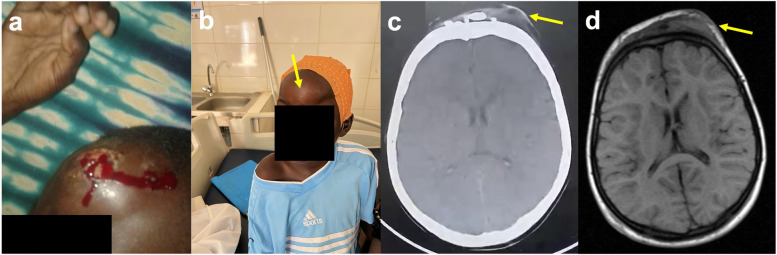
Preoperative findings. a Traumatic forehead injury sustained during drop attacks. b Forehead chronic traumatic scalp lesions resulted from repeated head impacts during drop attacks (yellow arrow). c CT demonstrated prominent forehead callosities (yellow arrow) without underlying structural lesion. d MRI showed forehead lesions (yellow arrow) but otherwise confirming the absence of focal pathology
